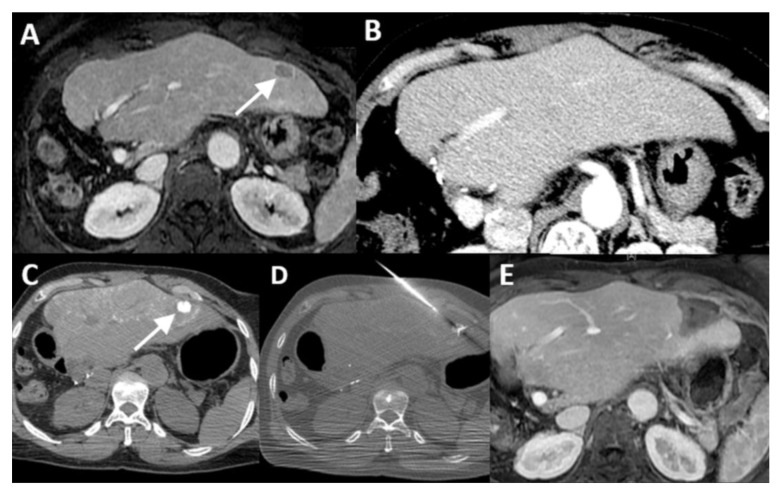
Visualization of the lesion by Lipiodol^®^ Ultra Fluid contrast agent. Solitary colorectal liver metastasis (arrow) in the left liver lobe is visible on diagnostic MRI (A), but not on the intraprocedural, intravenous contrast-enhanced CT in the late arterial to early portal venous phase (B). After selective catheterization of the left hepatic artery and administration of intra-arterial Lipiodol^®^ Ultra Fluid contrast agent (Guerbet, Villepinte, France), the lesion (arrow) became clearly visible as an enhancing nodule on non-enhanced CT imaging (C). Percutaneous RFA (Cool-tip™, Medtronic-Covidien, Boulder, CO, USA) was performed successfully (D). Follow-up imaging showed no signs of local tumor progression (E).
